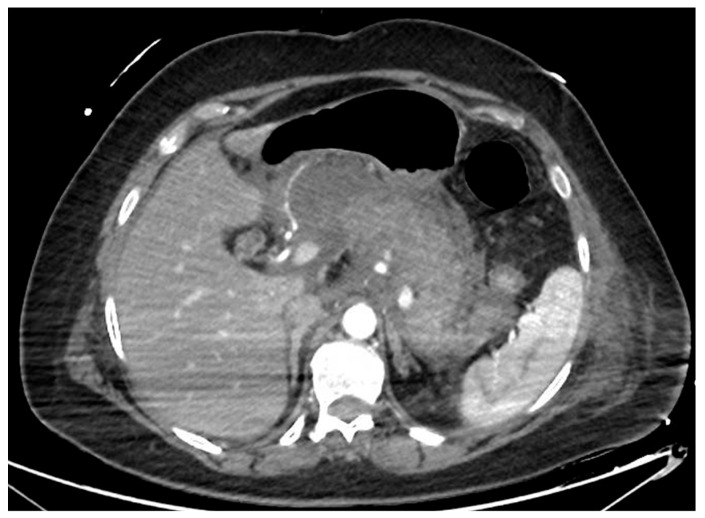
CT scan image of a necrotizing pancreatitis, with hypoenhancing areas of pancreatic parenchyma. CT, computed tomography.
